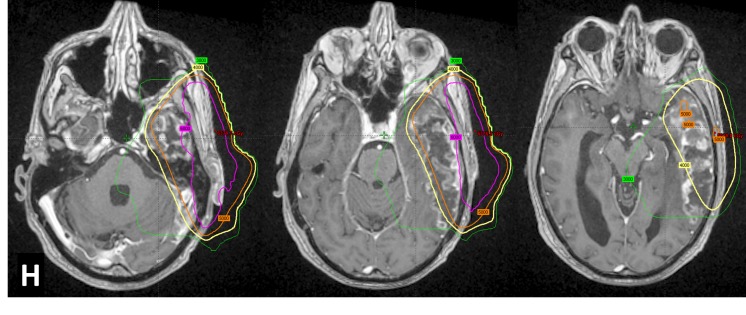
Super-imposition of treatment planning isodoses on the axial post contrast MRI images H- Extent of temporal lobe necrosis following the 40 Gy isodose (yellow), 60 Gy isodose confines to the extracranial tissue (pink) and the lesion mostly encompassed by the 50 Gy isodose (orange).
